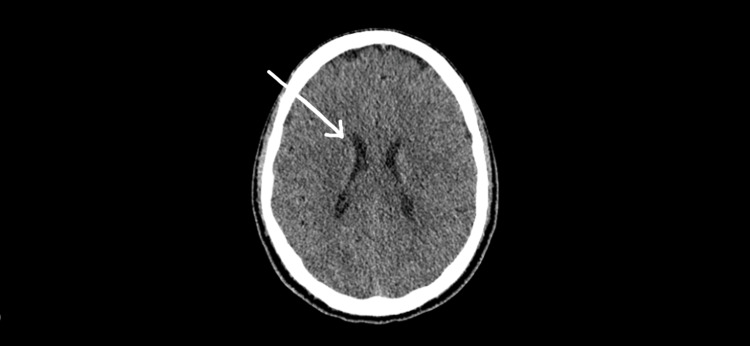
Axial CT brain showing a very mild ventricular dilatation, straight midline, and no pathology CT: computed tomography
